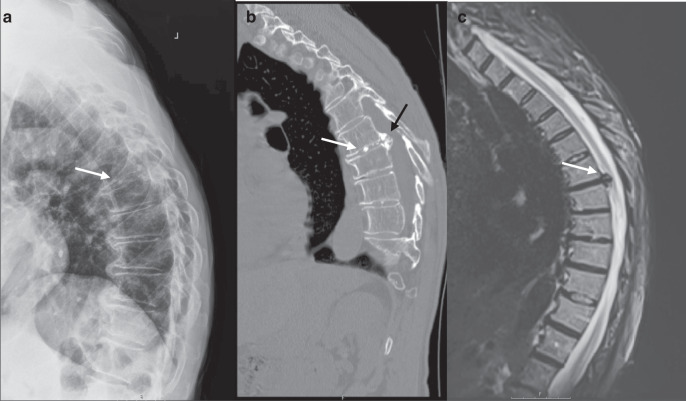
Clinical presentation of disc calcification - X-ray and MRI images. 72-year-old male who presented with a calcified thoracic disc herniation for pre-surgical workup. a Preoperative lateral view x-ray demonstrating intervertebral disc calcification (arrow) in the thoracic spine. b CT sagittal reconstruction of thoracic spine demonstrating disc calcification (white arrow) and herniation of calcified material extending posteriorly (black arrow). c Sagittal T2-weighted MRI demonstrating disc herniation into the spinal canal (white arrow); calcified material within the disc space is not clearly visualized
